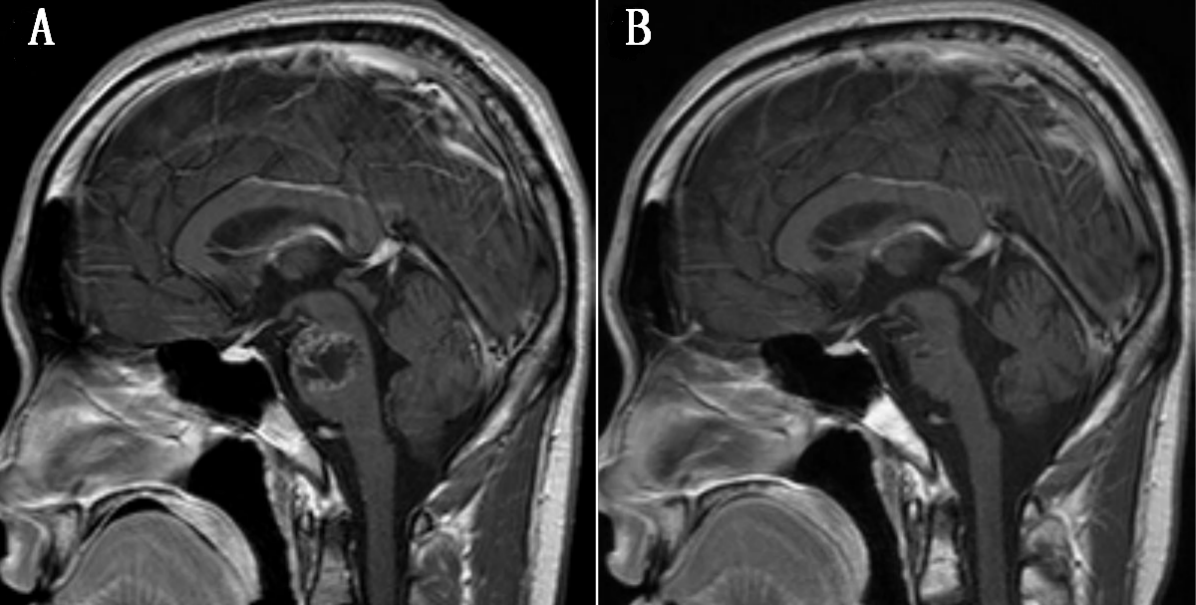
Nine-month after the first operation, magnetic resonance imaging revealed a local recurrence of primary tumor, which showed heterogeneous enhancement on post contrast imaging (A). Two-year after secondary surgery plus radiotherapy. T1-weighted enhanced magnetic resonance imaging shows no evidence of disease residue or recurrence (B).
